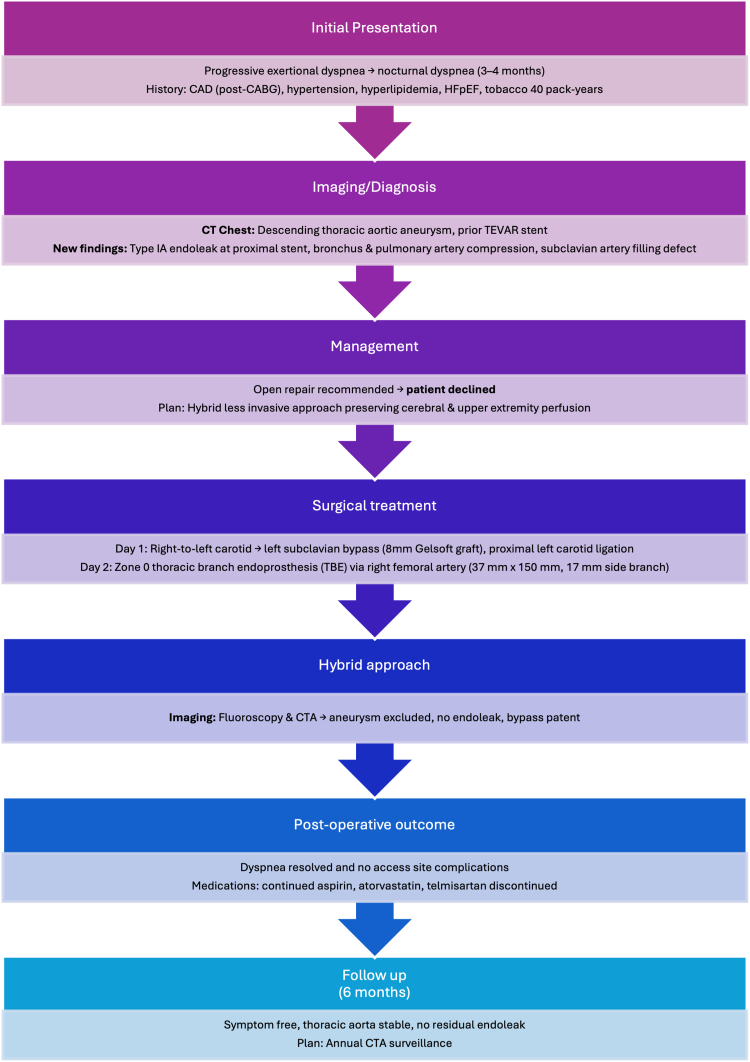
Timeline of Events Visual Summary CABG = coronary artery bypass graft; CAD = coronary artery disease; CTA = computed tomography angiography; HFpEF = Heart failure preserved ejection fraction; TEVAR = thoracic endovascular aortic repair.
